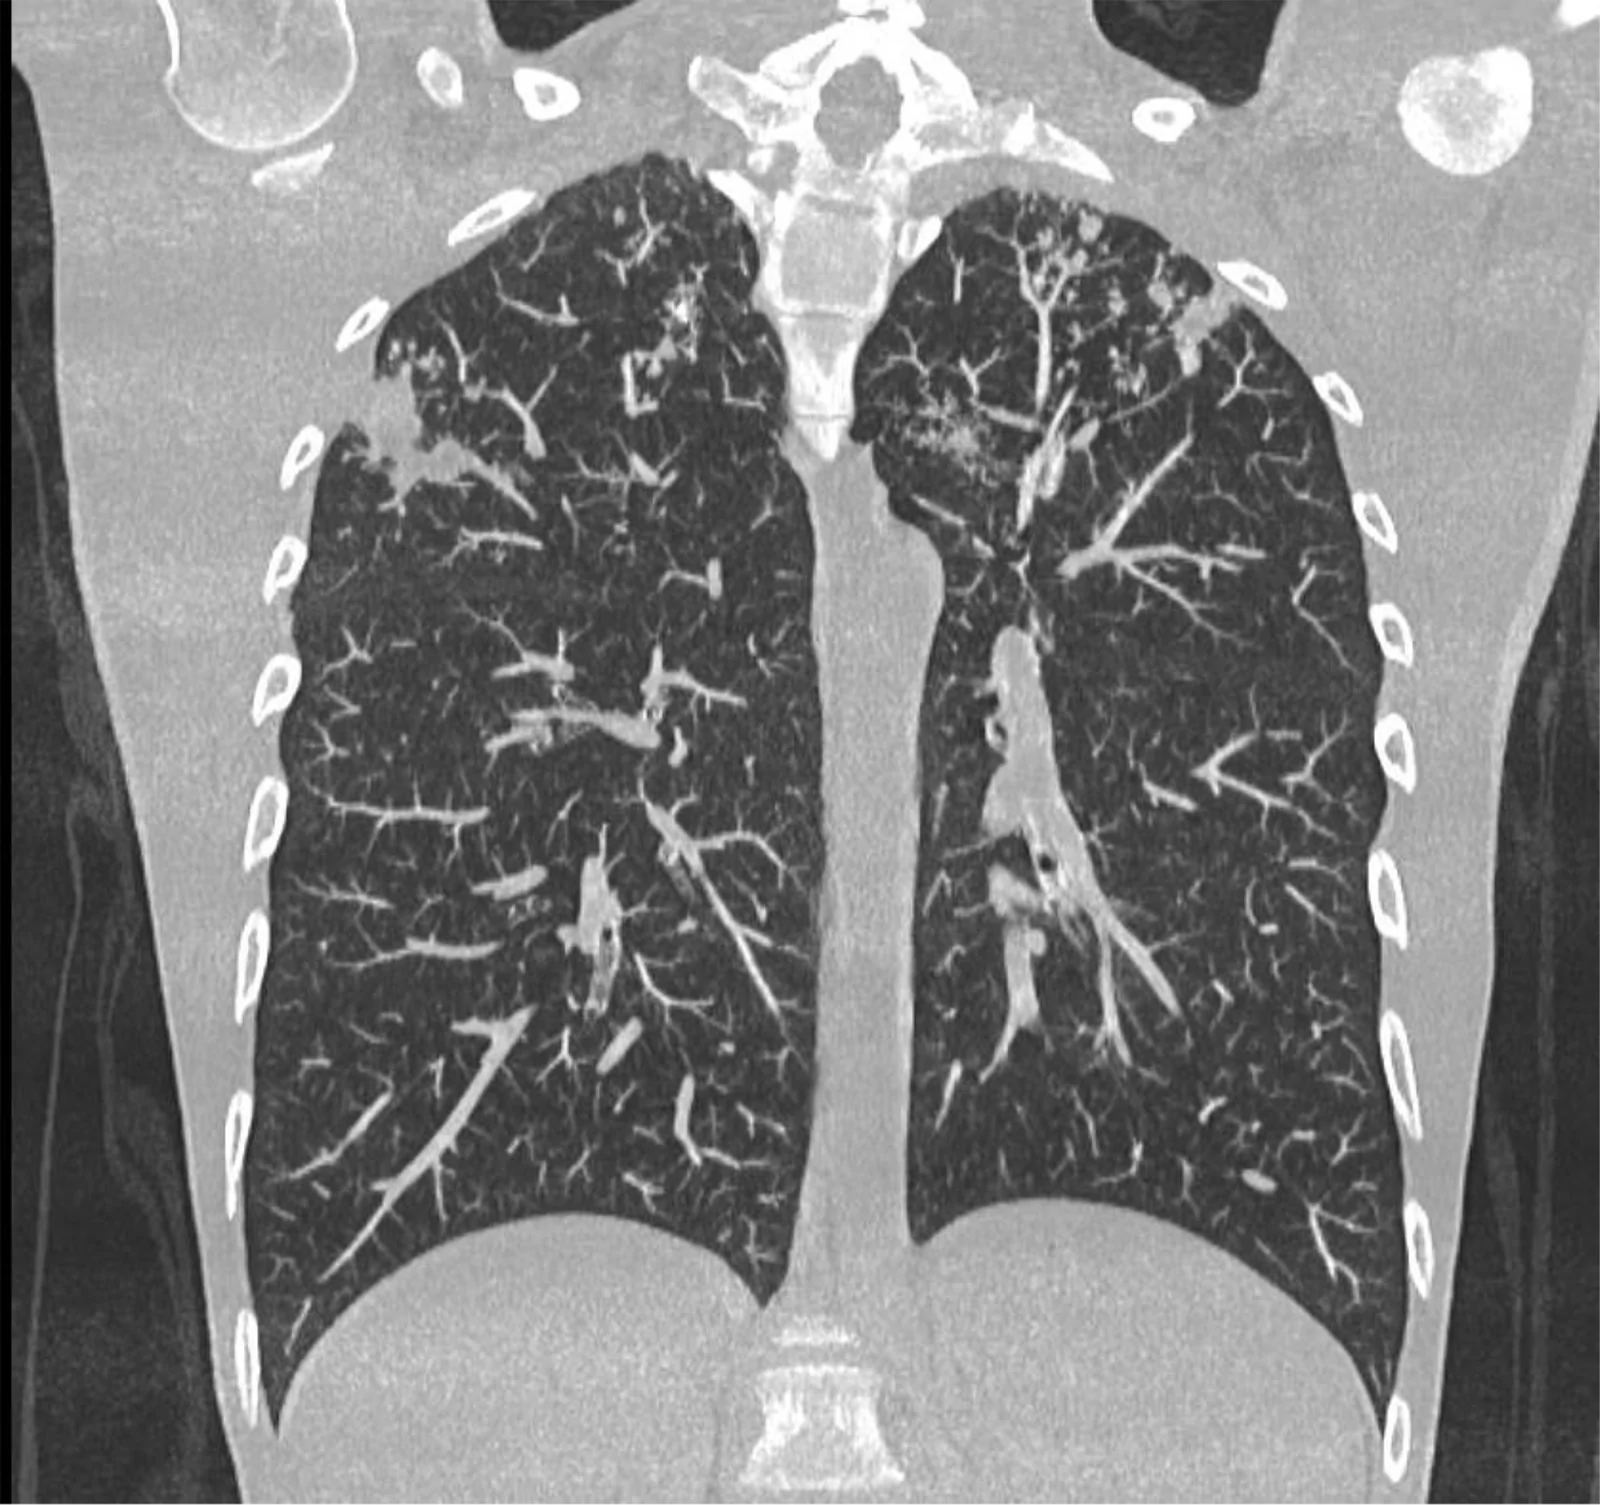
Coronal CT scan of the chest in parenchymal window with maximum intensity projection reconstruction: multiple bilateral micronodules in the upper lobes with a branching distribution, giving a ‘tree-in-bud’ appearance.
